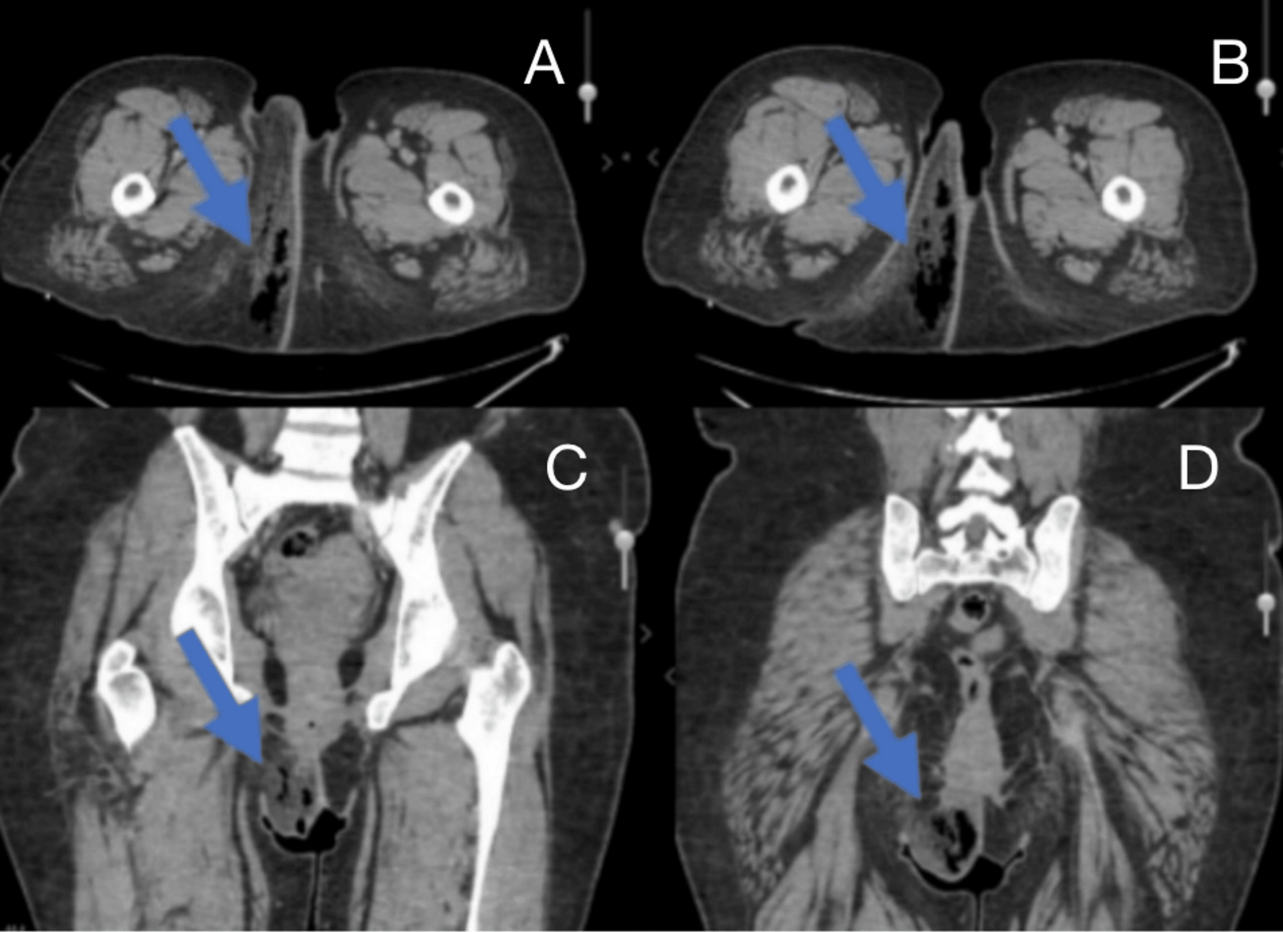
Patient's computed tomography scan Computed tomography (CT) images demonstrating soft tissue changes in the perineal and vulvar regions. (A) Transverse CT scan, cephalad level, showing right labial induration and subcutaneous fat stranding (arrow). (B) Transverse CT scan, more caudal section, showing progression of inflammation into the perineal region (arrow). (C) Coronal CT scan demonstrating involvement of the right labia majora and perineum (arrow). (D) Coronal CT scan showing inferior extension of inflammatory changes toward the posterior perineum (arrow).
